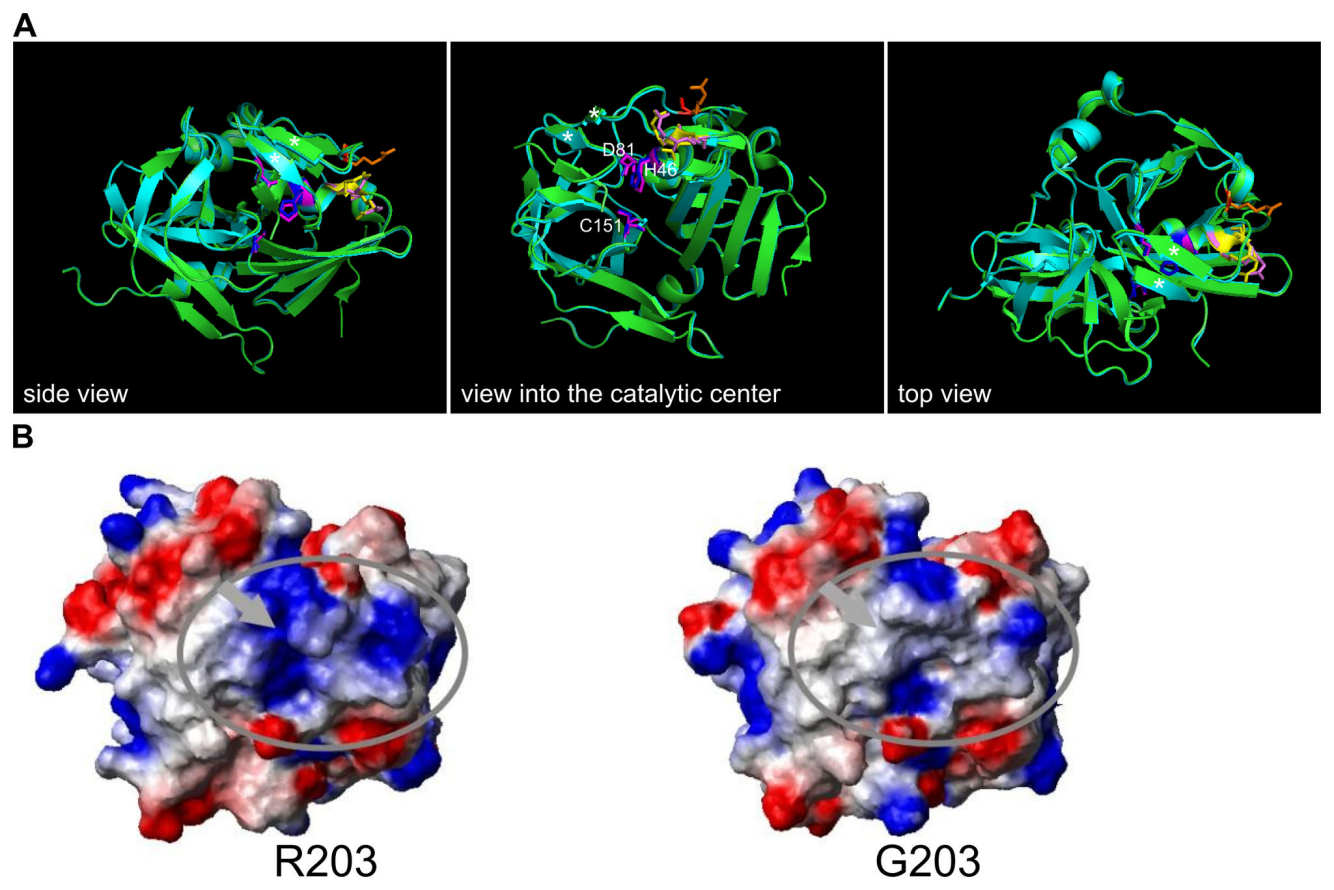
Structural comparison of the TEV protease with the R203G mutant. A) Ribbon structure of the TEV protease (green) was overlaid with the mutant (dark cyan). The structure of the R203G mutant, which corresponds to R345G in the pTEV^+^ protease, was obtained by homology modeling using an x-ray structure of the TEV protease as template. Views from three different sides are shown. The residues of the catalytic triad H46, D81, and C151 are indicated (TEV protease: blue; mutant: magenta). The two arginine residues close to the catalytic center (R49, R50) are shown in yellow (TEV protease) and light magenta (G203 mutant). The R203 residue is shown in orange, the G203 in red. The two β-sheets, which are mentioned in the text that close the catalytic center are marked by asterisks. B) Surface charge distribution of the TEV protease compared to the R203G mutant. Surface charges were calculated using the software package MolMol. Positive charge is represented by blue color, negative charge by red color.
